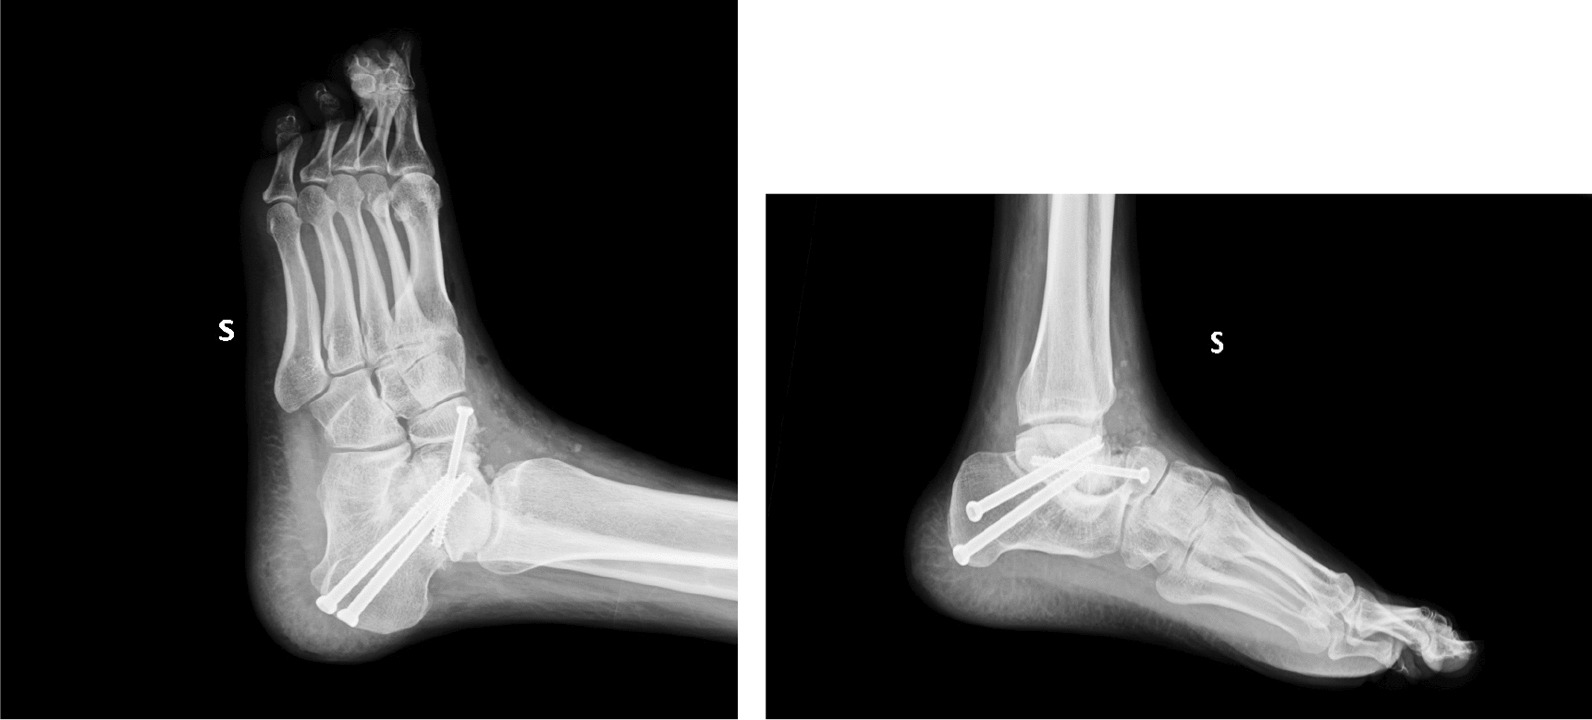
Post-operative X-Ray after surgery
